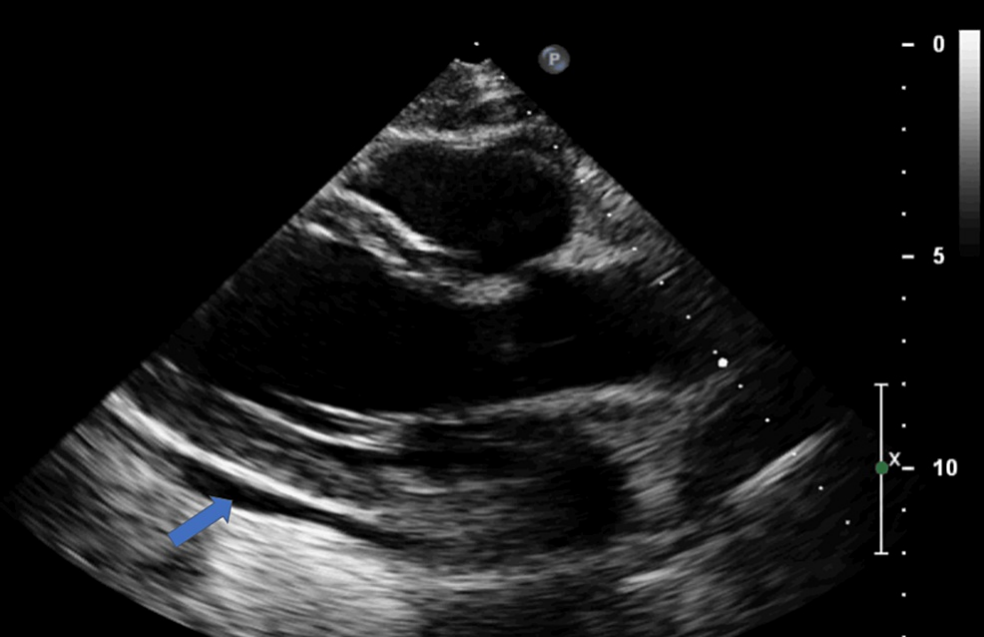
Transthoracic echocardiogram showing small pericardial effusion around the lateral wall of the left ventricle (blue arrow).
